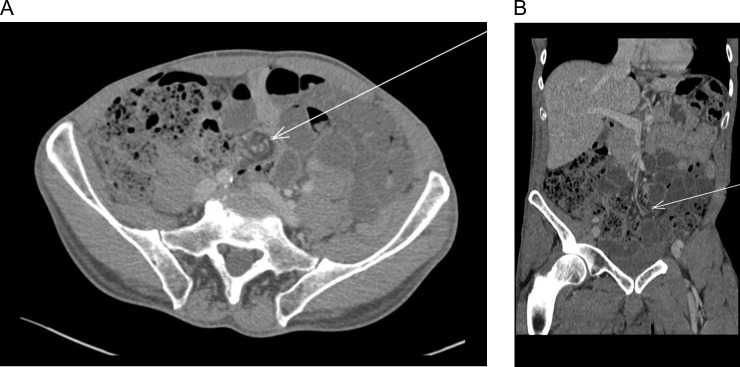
(A) Abdominal computed tomography (axial) revealed dilation of small intestine with a rotation of the mesentery around the mesenteric vessels (whirl sign) (arrow). (B) Abdominal computed tomography (coronal) revealed dilation of small intestine with a rotation of the mesentery around the mesenteric vessels (whirl sign) (arrow).
